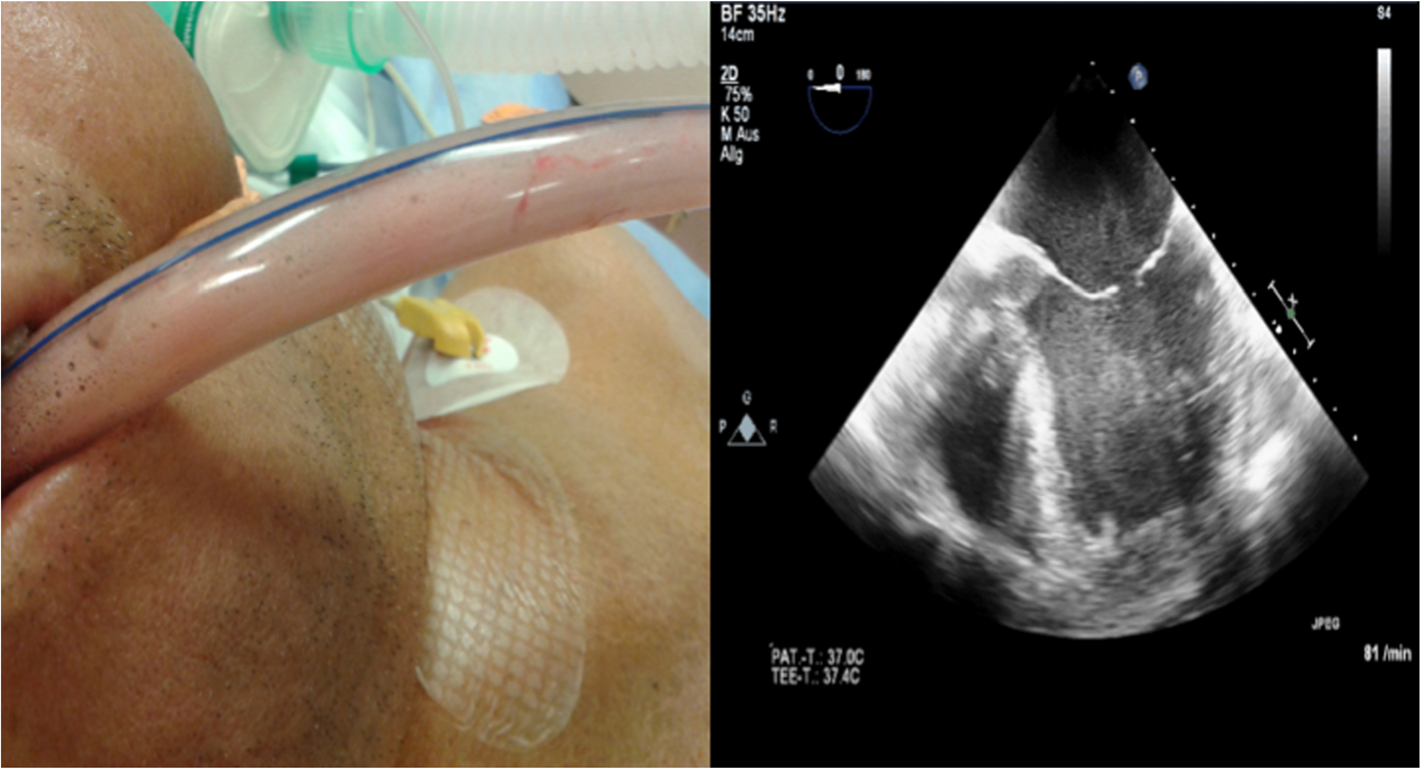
Clinical images from a representative patient of our study cohort: Left – Endotracheal tube with overflowing secretions, Right – Transesophageal echocardiography demonstrating dilated left ventricle with stasis despite peripheral ECMO support (CardioHelp™-System, Maquet, Rastatt, Germany) on admission.
